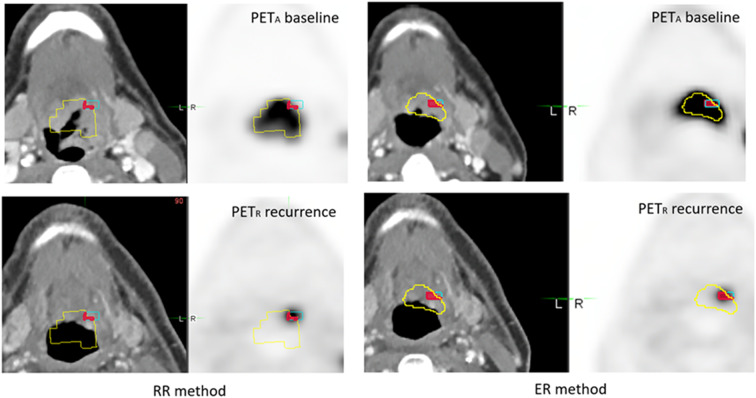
Example of 49 year-old man with an T4N2M0 oropharyngeal HNSC. During routine clinical monitoring, a FDG-PET/CT scan was performed 7 months after chemoradiotherapy, and demonstrated an occult local relapse. PET_A_ (images on the top) and PET_R_ (images on the bottom) were scanned in treatment position (TP) and coregistered with RR method (left) and ER method (right). A_40_ subvolume is represented as a yellow line, R_40_ subvolume as a blue line and A_40_∩R_40_ in red area. The OF (A_40_, R_40_) index was calculated respectively at 0.19 and 0.64 for RR and ER method.
